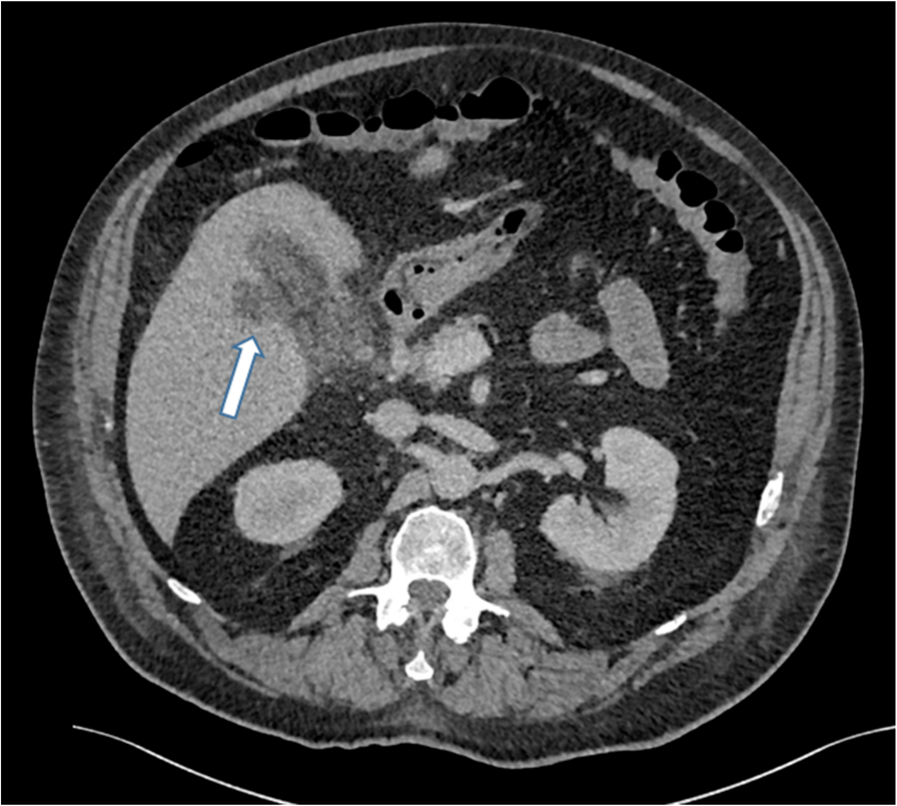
Axial contrast-enhanced CT of the abdomen. There is gallbladder wall thickening and pericholecystic fluid consistent with acute cholecystitis. There is a defect in the medial gallbladder wall with a hypoattenuating collection within segment 5 of the liver (arrow). Appearances are consistent with gallbladder perforation and hepatic abscess
